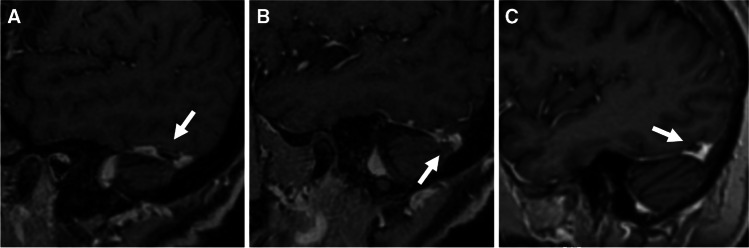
MR imaging example of BHAG compared to “simple” arachnoid granulation. A Sagittal T1 post-contrast MR images of patient 11 (right-sided PT) show left occipital BHAG into the transverse sinus, as well as B right cerebellar BHAG into the transverse sinus in the absence of IIH. C Sagittal T1 post-contrast MR image of a separate patient demonstrates an arachnoid granulation in a similar location without brain herniation
